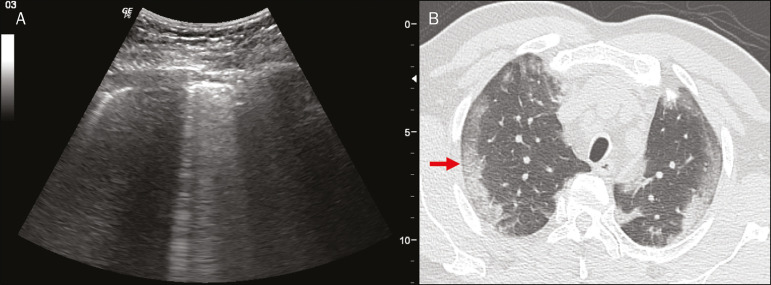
Chest ultrasound and CT in a patient with COVID-19: correlation between the findings. A: Coalescent B-lines characterized by the white lung appearance on ultrasound. B: On CT, peripheral ground-glass opacities (arrow) are identified at the same site.
